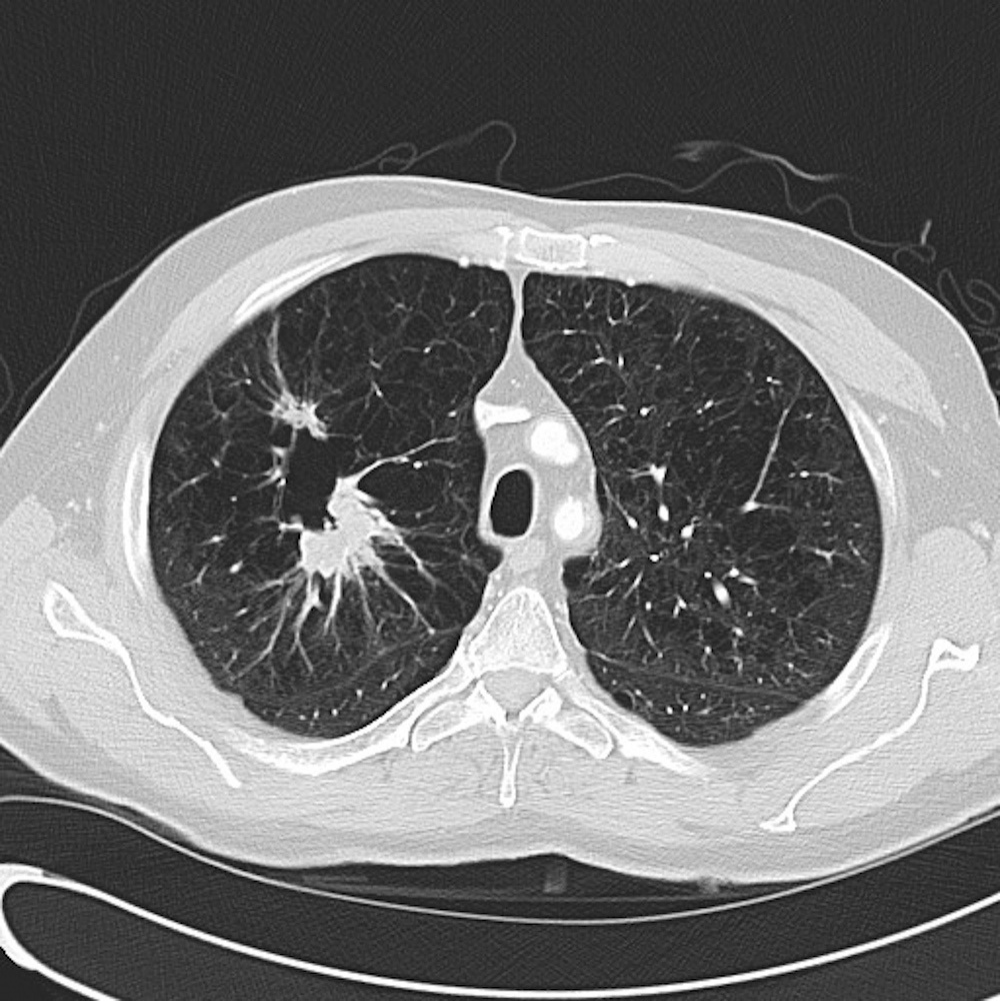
CT scan of the chest demonstrated two spiculated masses in the right upper lobe of the lung.
